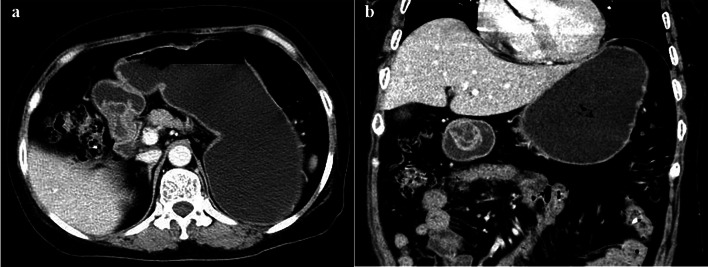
61-year-old woman with GCP showing a 3.3-cm cystic mass. Axial venous phase (a) and coronal venous phase (b) CT images show the cystic mass located in the gastric antrum. This lesion is featured as multiple cysts separated by fibrous
